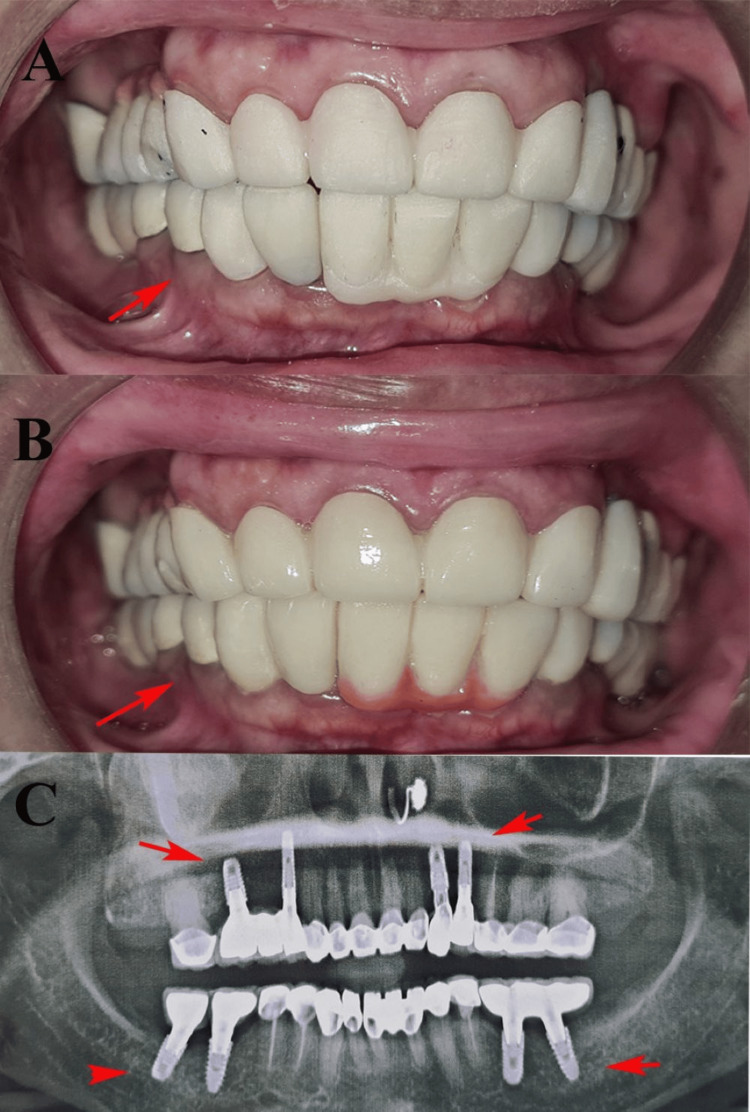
(A) Bisque try-in for maxillary and mandibular teeth. (B) Polished metal-ceramic maxillary and mandibular implant-supported hybrid bridge. (C) Postoperative follow-up orthopantomogram (OPG) after three months. These images are related to our patient and published with the patient's consent.
